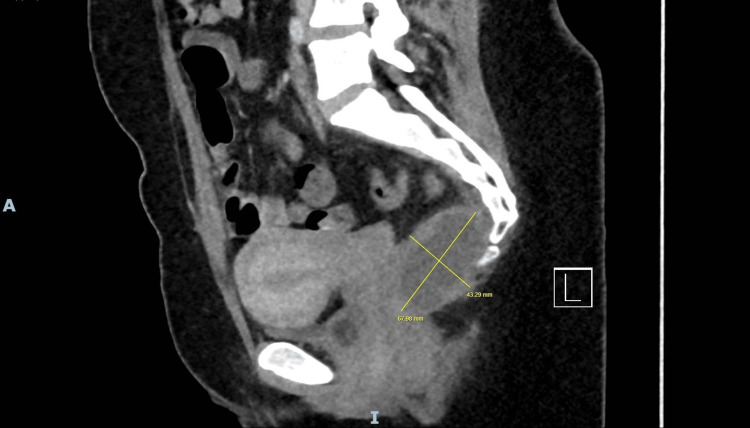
Sagittal CT pelvis (2025) demonstrating progressive enlargement of presacral cystic lesion extending into ischiorectal fossa
